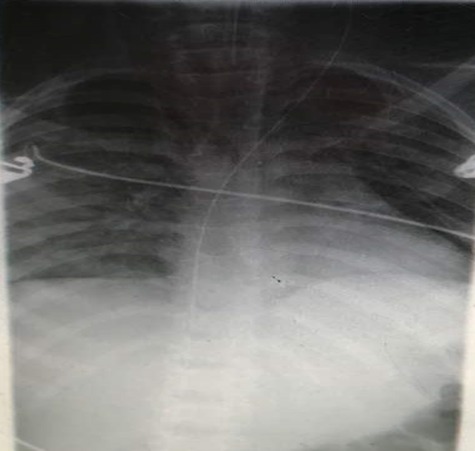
An anteroposterior chest x-ray showing the path of the guidewire through the inferior vena cava, right atrium, the superior vena cava, the innominate vein and into the left internal Jugular vein
